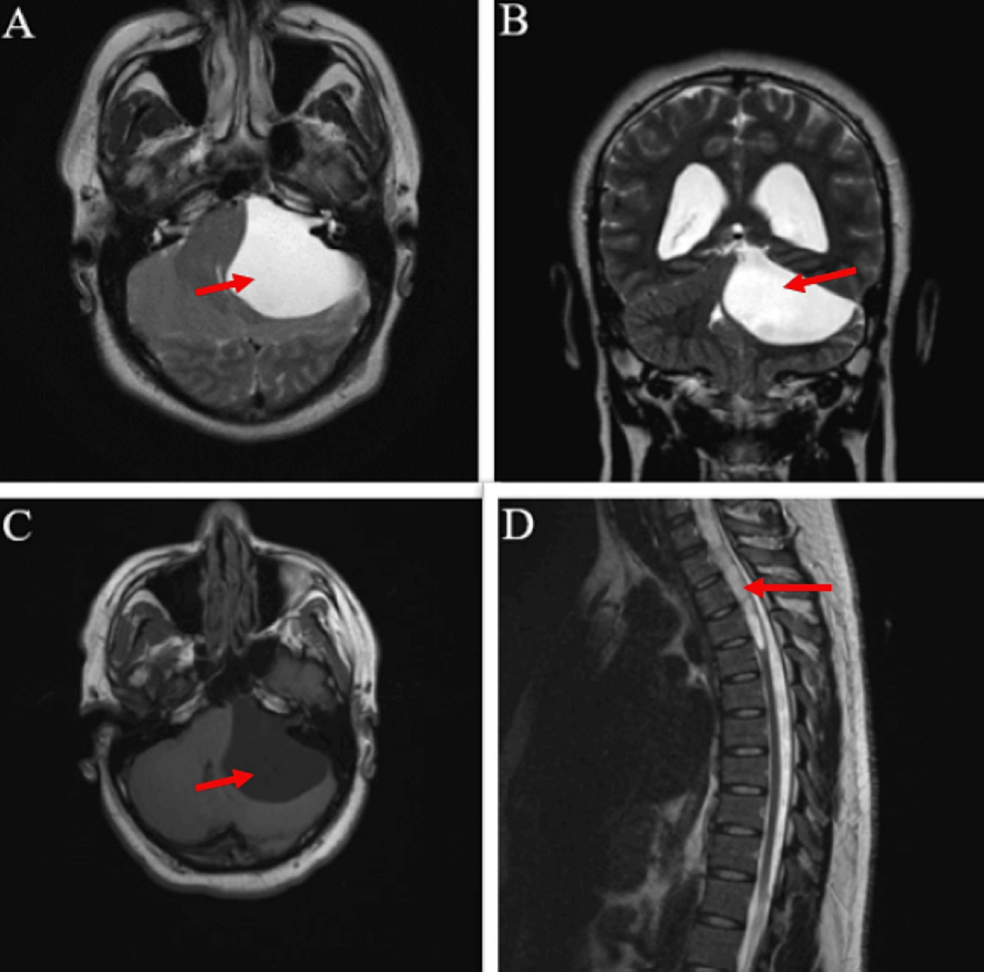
Preoperative MRI with arrows demonstrating the PFAC at the left CPA with mass effect on the midbrain, pons, and cerebellum (a) axial T2; (b) coronal T2; (c) axial T1; (d) sagittal T2 with arrow demonstrating syringomyelia
